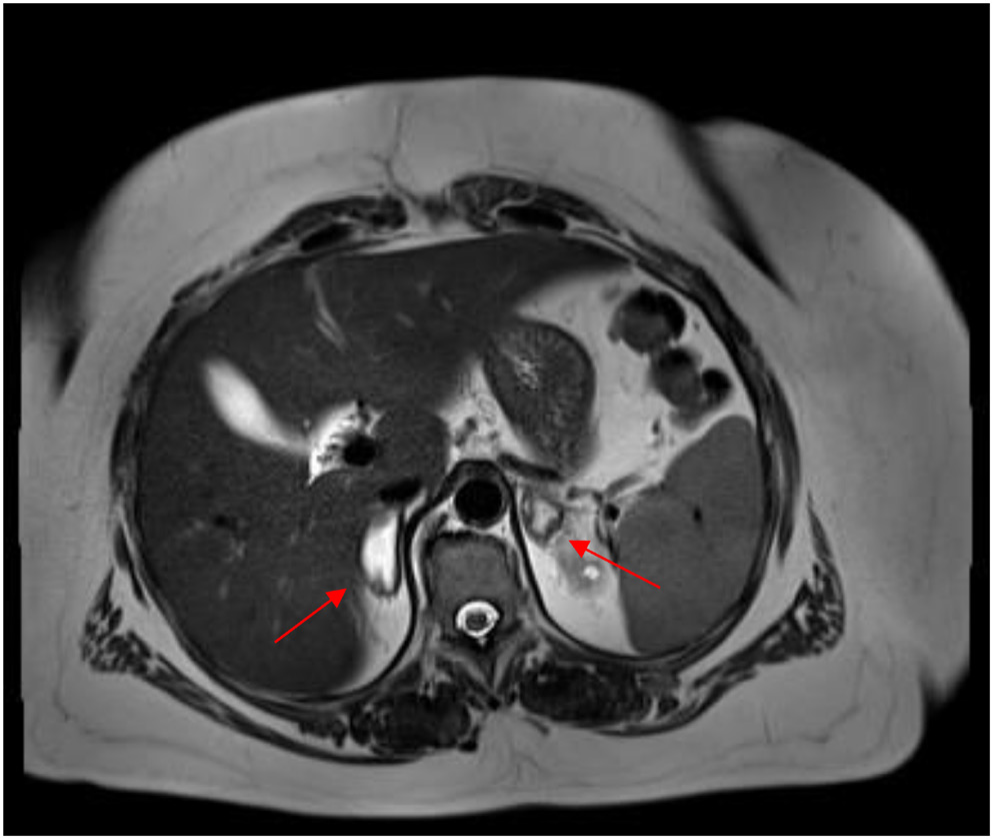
MRI abdomen in transverse plane showing bilateral hemorrhage in the adrenal glands (arrows) on a T2-weighted image.
